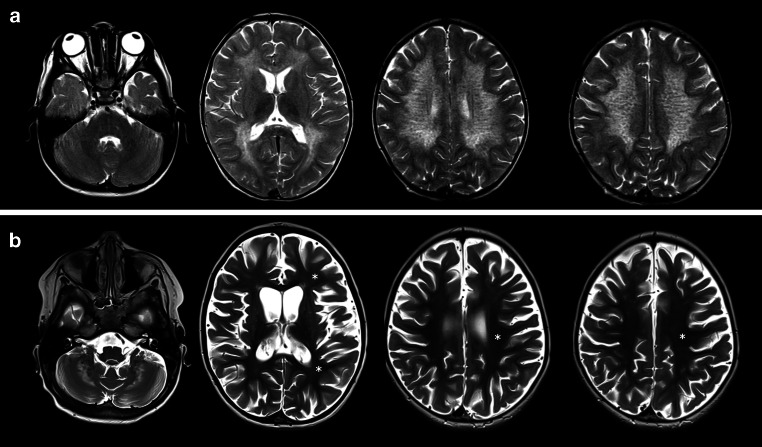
Example for T2-pseudonormalization: characteristic T2-hyperintensities of white matter (WM) are shown in the MRI of one exemplary patient at the time of diagnosis with the age of 2.8 years (a). In a follow-up scan at the late stage of the disease with 4.4 years (b), T2 hyperintensities of supratentorial WM are barely evident aside from WM in the cerebellum. *Asterisk* T2-pseudonormalization
